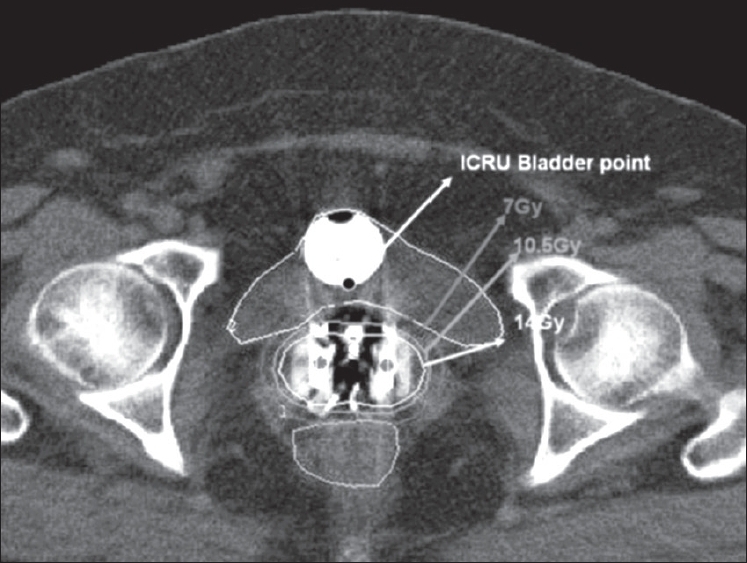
Dose distribution of image-based CT planning for a representative patient
